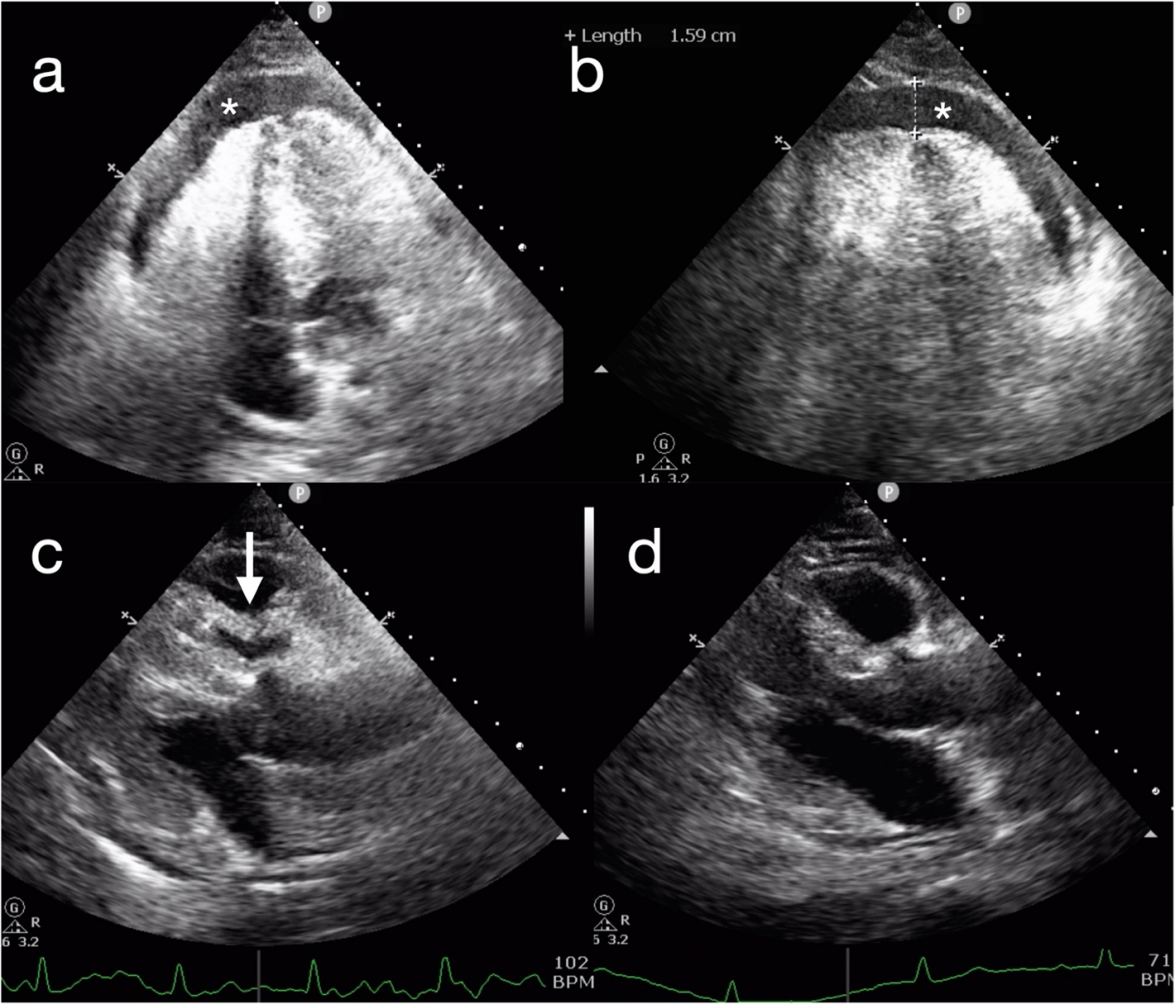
Echocardiography with a four-chamber view and a parasternal long axis. Circumferential pericardial effusion (*) (Panel a) was 1.59 cm in the largest dimension (Panel b). Sign of cardiac tamponade (Panel c): diastolic collapse of the right ventricle with pericardial effusion (white arrow). The pericardial effusion disappeared after the pericardiocentesis (Panel d)
